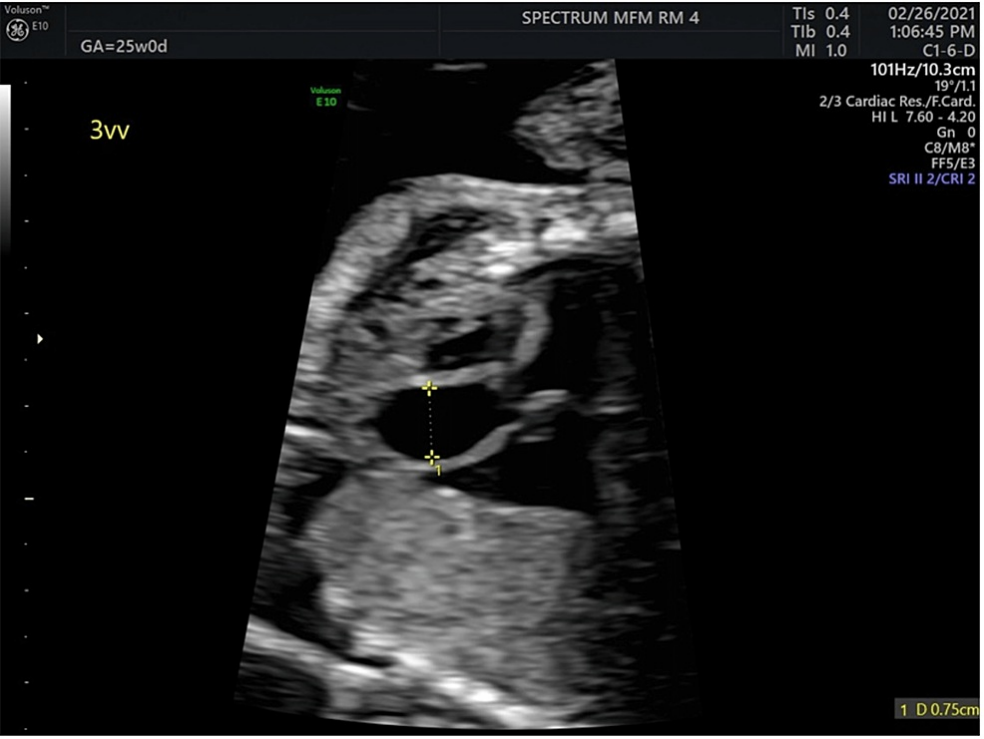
Initial echocardiogram at 25 weeks of gestational age Left ventricular outflow tract (LVOT) view demonstrating a dilated ascending aorta diameter measuring 7.99 mm, immediately distal to the LVOT at the initial echocardiogram at 25 weeks of gestation
